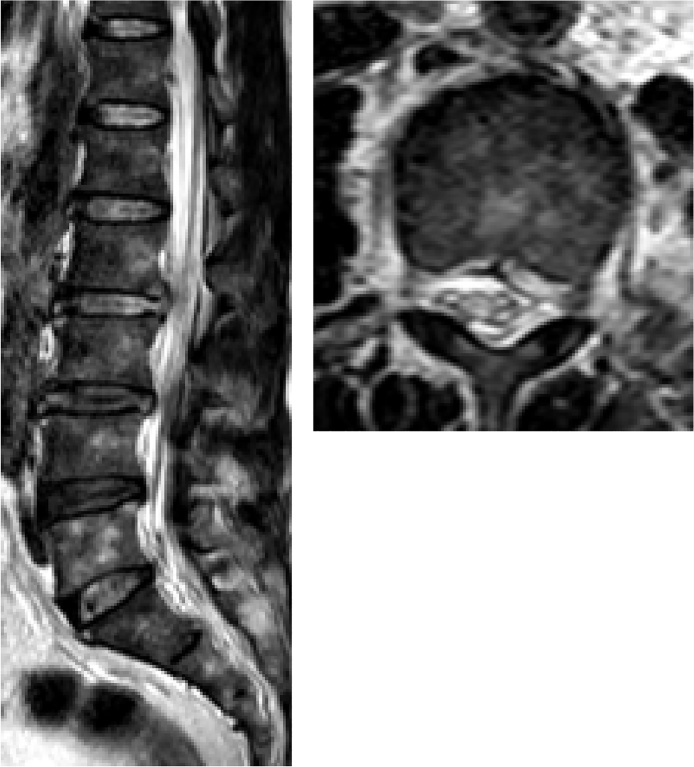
The postoperative MRI demonstrated the disc fragment was removed and left nerve root was decompressed.
